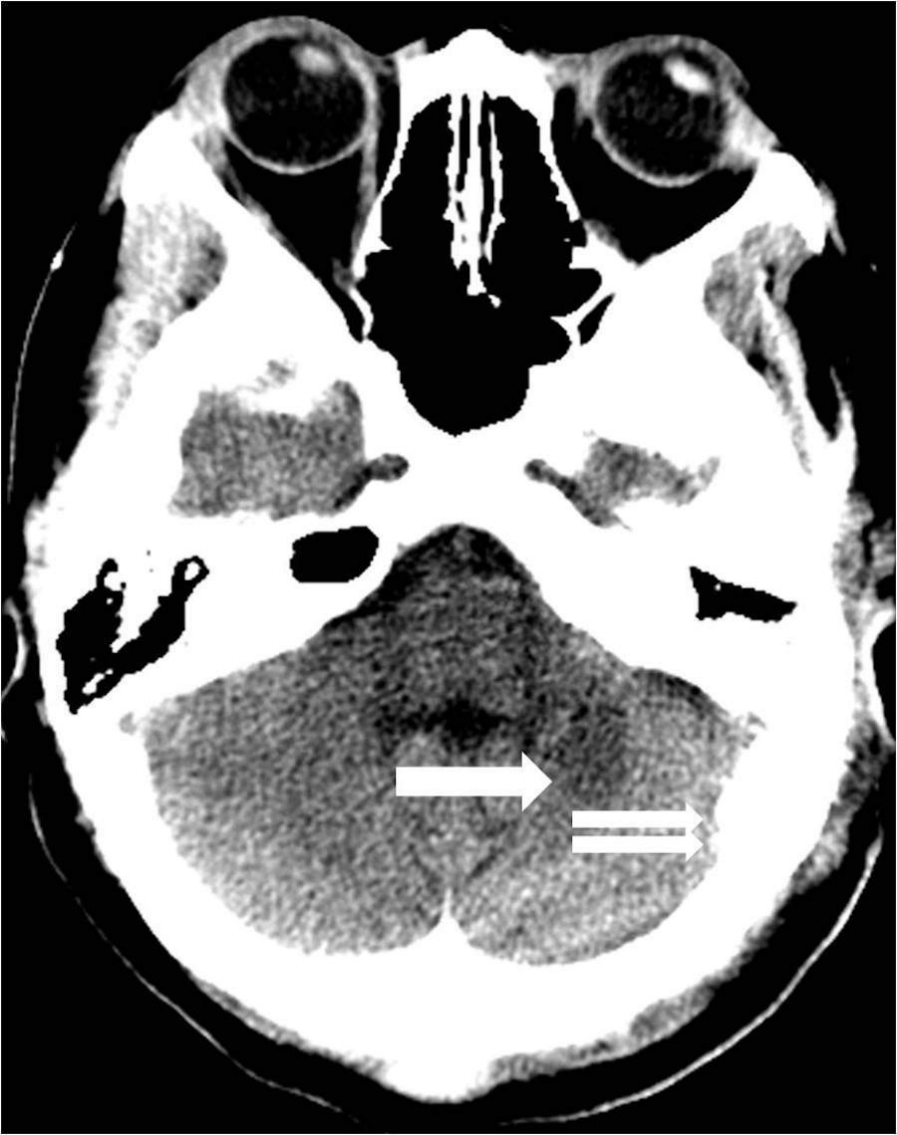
Postoperative head computed tomography two weeks after surgery reveals a focal area of encephalomalacia where the hematoma used to reside (arrow). Subtle changes from a left retromastoid craniotomy are evident (double arrow).
